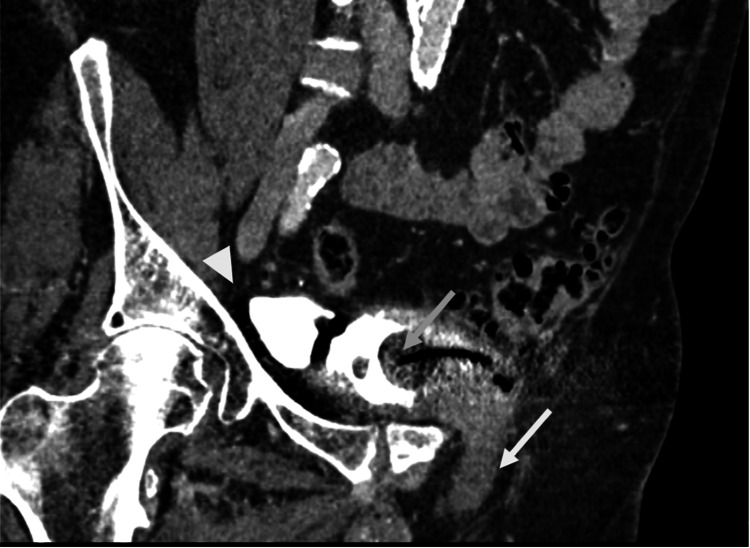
Oblique coronal reformats of the pelvis on CT urogram show a Foley catheter balloon in the bladder (gray arrow), a bladder diverticulum filled with contrast on the patient’s right (white arrowhead), and the urine-filled bladder hernia extending into the left inguinal canal (white arrow)
